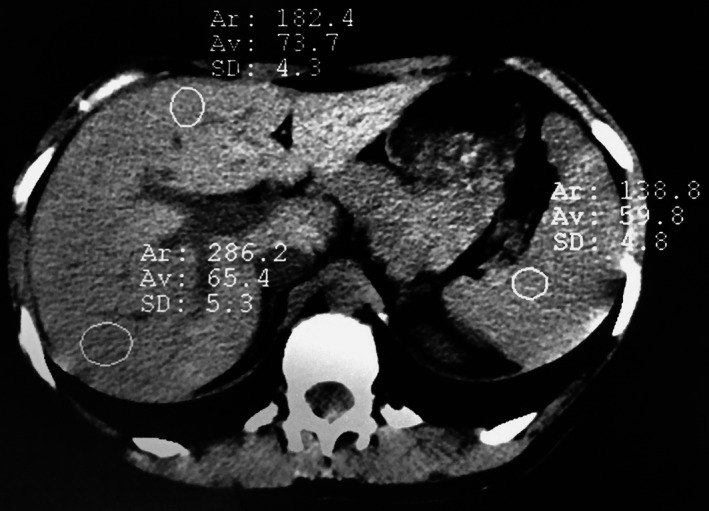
CT 7 days after delivery : showed improvement of lesions.
